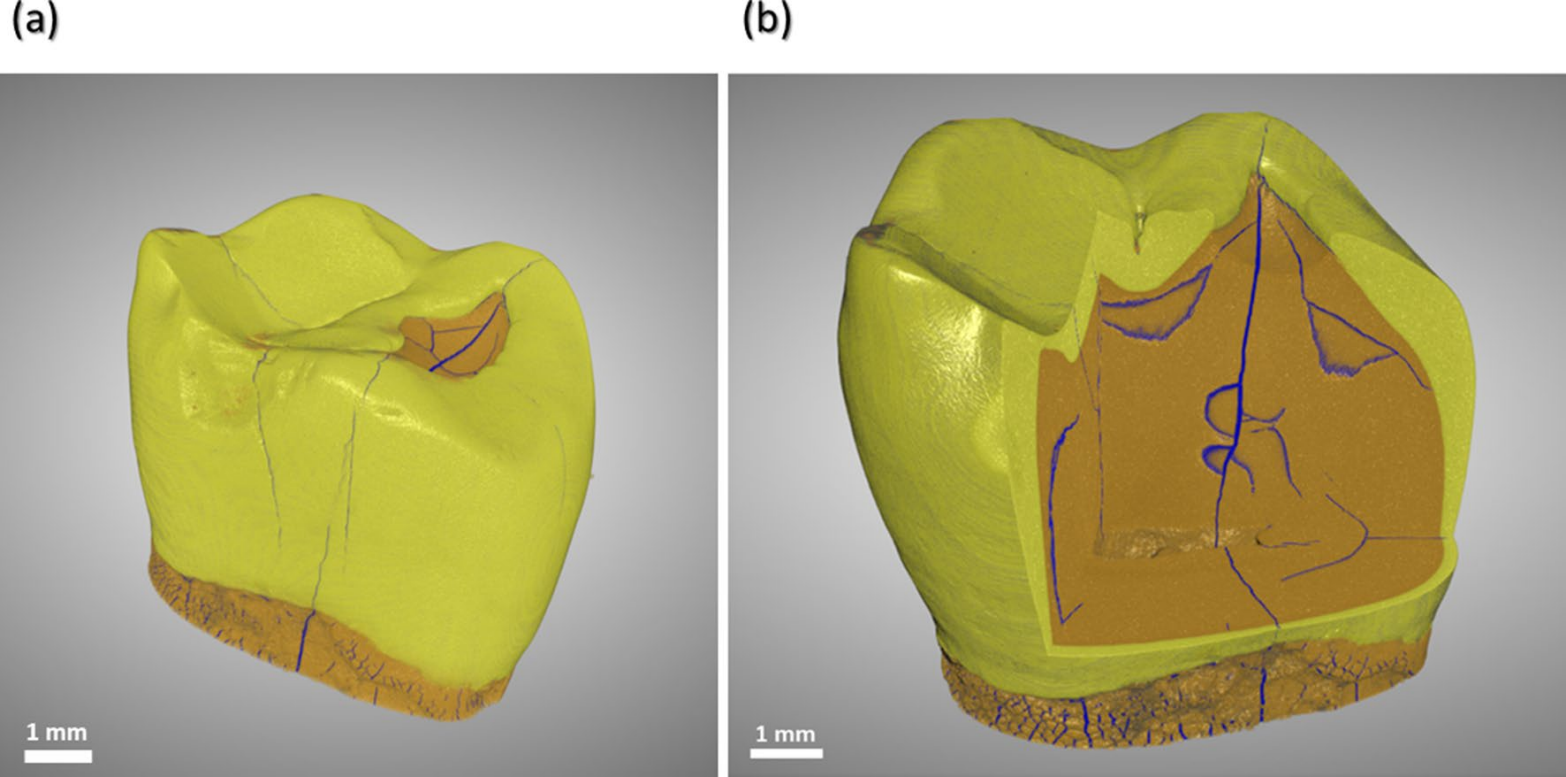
Scanned image (a) of the entire tooth using X-ray $$\mu $$CT and (b) a 3D virtual cut through a segmented tooth showing cracks (blue) inside the enamel (yellow) and the dentin (brown; see Supplementary Video S1).
